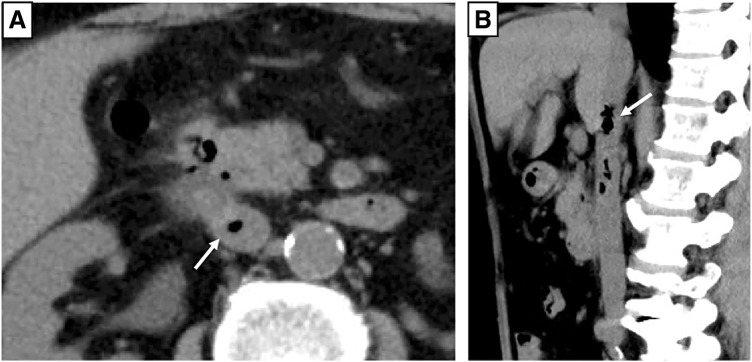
CT scan when the patient complained of abdominal pain. A white arrow in both axial (A) and sagittal (B) CT scans indicates an air bubble in the inferior vena cava. CT, computed tomography
